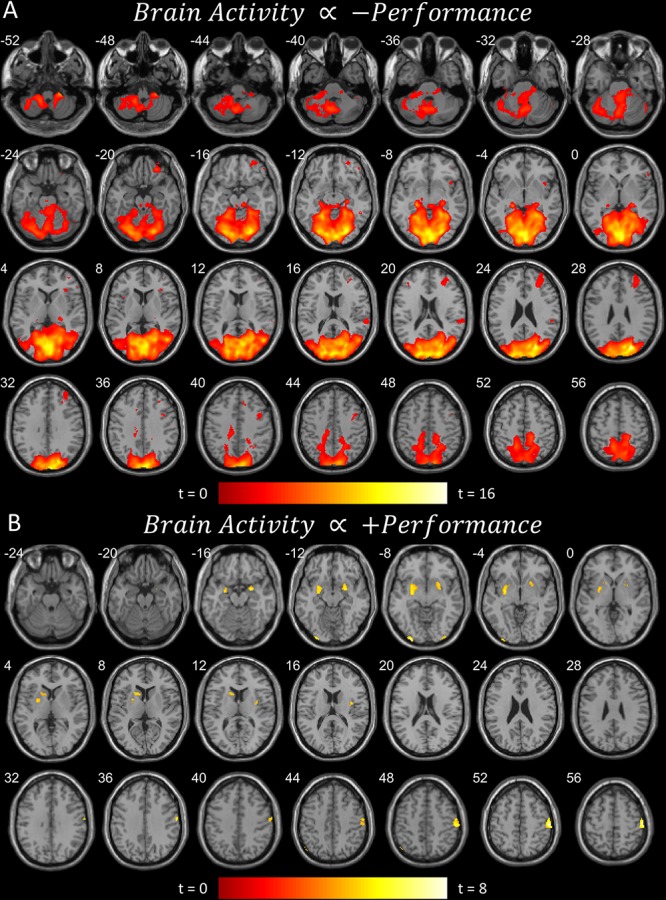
(A) Brain areas that become more active when performance worsens, or less active when performance improves (pFDR < 0.05 peak level corrected across entire brain) rendered on anatomical MRI slices using xjView toolbox (https://www.alivelearn.net/xjview). Note cerebellar and cortical activity. (B) Brain areas that become more active when performance improves, or less active when performance worsens. Same rendering as (A). Note basal ganglia activity.
